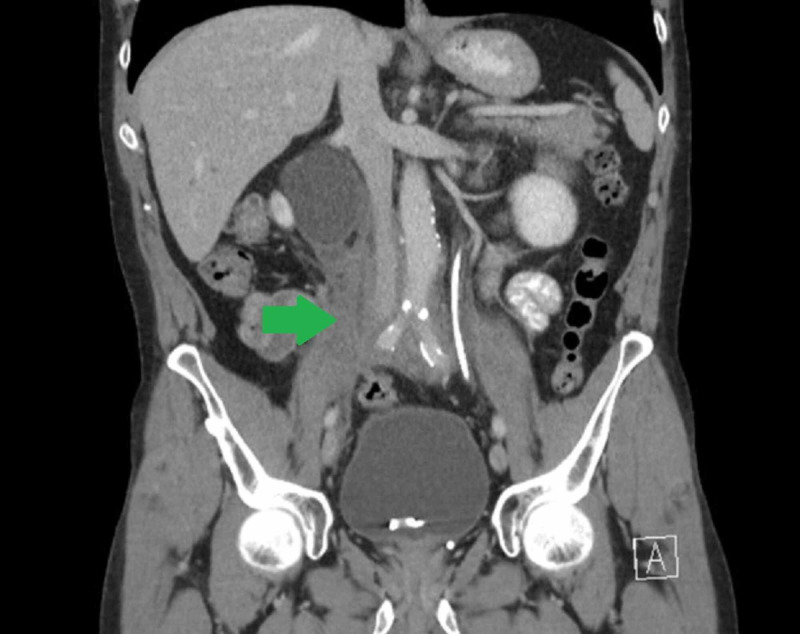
Follow-up abdominal CT scan. Decreased size (7.7 vs 8.1 cm) of the right-sided retroperitoneal mass.
